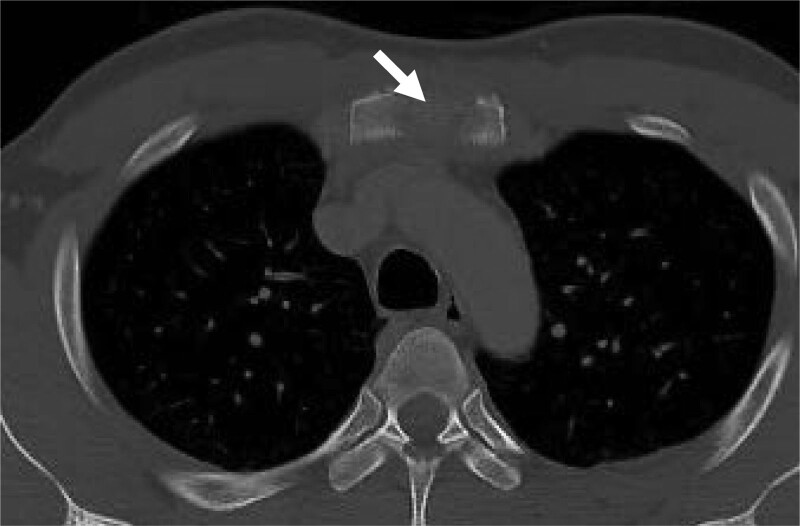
Osteolytic lesion in the sternum on plain computed tomography scan (white arrow).
